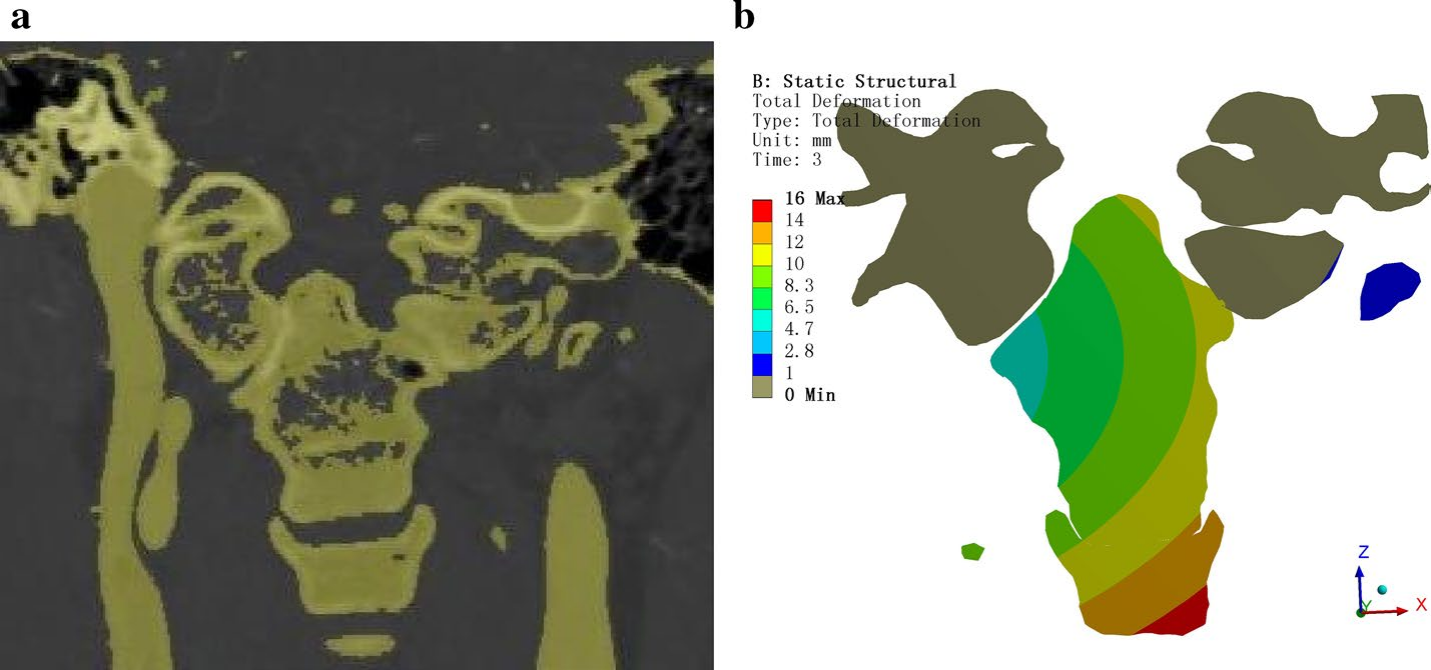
Comparison chart for the coronal plane. a CT. b ANSYS displacement cloud chart
